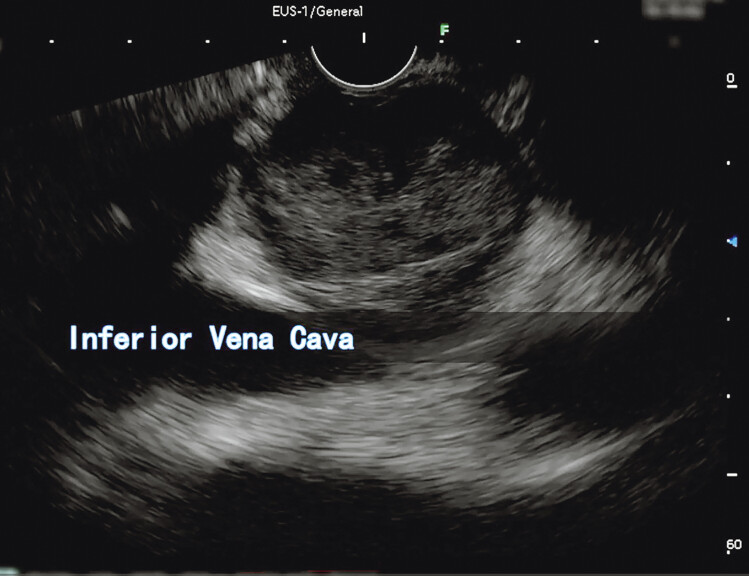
Endoscopic ultrasound showed a 7-cm hypoechoic mass originating from the inferior vena cava.
